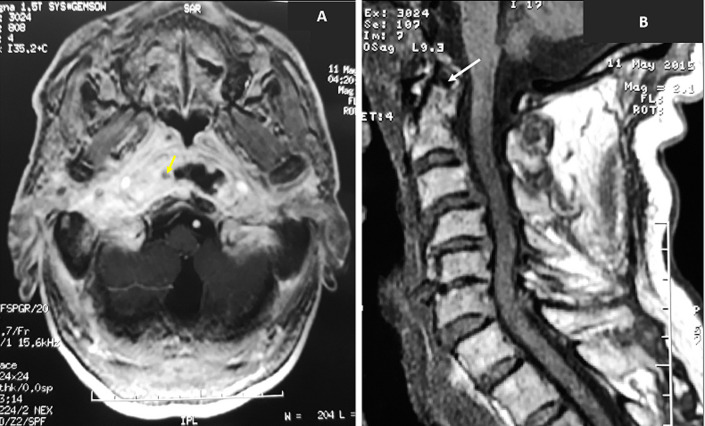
(A,B) temporal bone MRI of a patient with a right fungal NEO showing an extension laterally an abscess of the right parapharyngeal space (yellow arrow) and posteriorly to the atlo-axoidien joint (white arrow)
